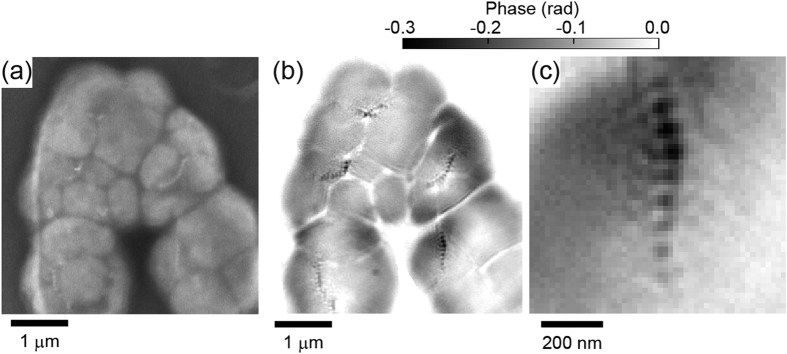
(a) SEM image of the magnetotactic bacteria MO-1. (b) Phase map of MO-1 obtained by dark-field X-ray ptychography. The pixel size is 17 nm. (c) Enlarged image of (b) showing the lower-right bacterium.
